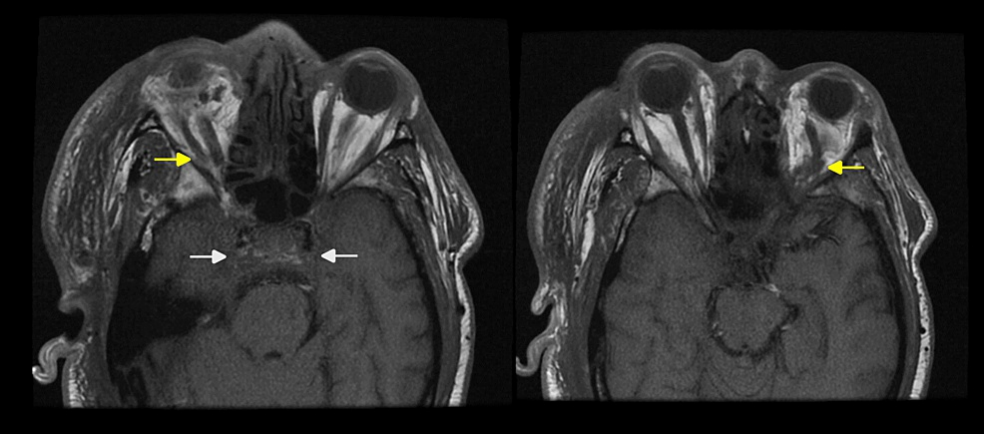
Axial T1 weighted MRI brain and orbits Axial T1-weighted MRI of the brain and orbits with contrast depicting bilateral cavernous sinus thrombosis (white arrows), bilateral superior ophthalmic vein thrombosis (yellow arrows), bilateral orbital cellulitis and proptosis with the right greater than the left, and right-sided pachymeningeal thickening and enhancement concerning for meningitis. MRI: magnetic resonance imaging
